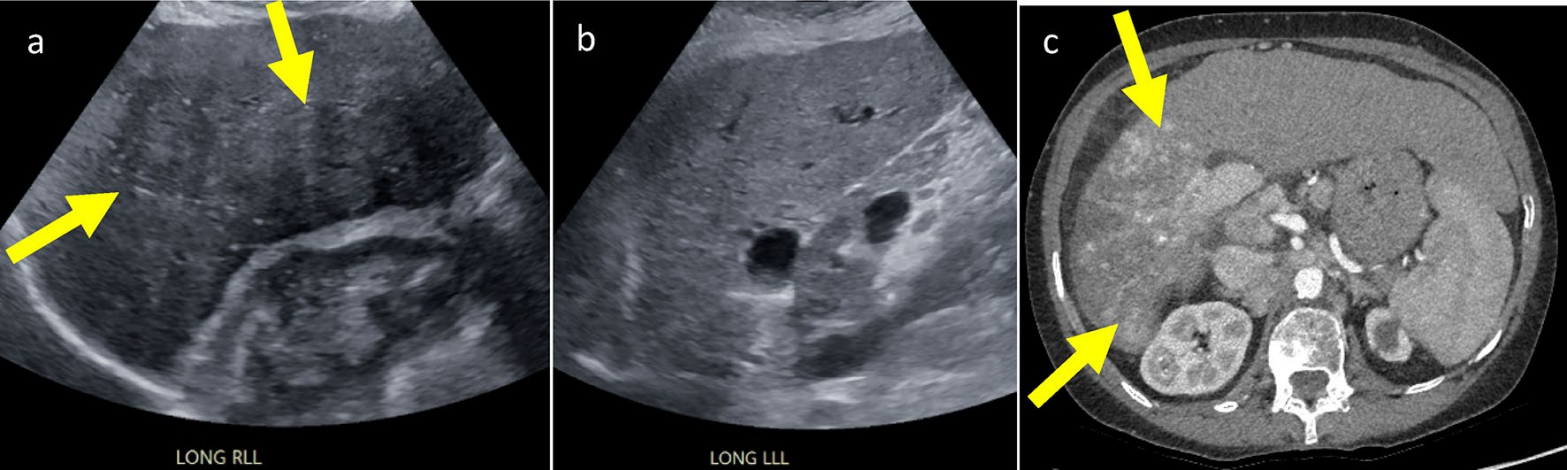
55-year-old female with ethanol cirrhosis and a 15 cm right lobe infiltrative cholangiocarcinoma not originally appreciated on US. Note that an infiltrative HCC could have a similar appearance. a Sagittal US image of the right lobe of the liver shows a large area of parenchymal distortion (arrows) with areas of refractive shadowing. b Sagittal US image of the left lobe of the liver, included for comparison, shows cirrhosis without parenchymal distortion. c Axial contrast enhanced arterial phase CT shows large area of heterogeneous enhancement (arrows) in the right lobe of the liver, corresponding to biopsy proven infiltrative cholangiocarcinoma. The right portal vein was not discretely visualized, presumably due to tumor in vein (not shown)
